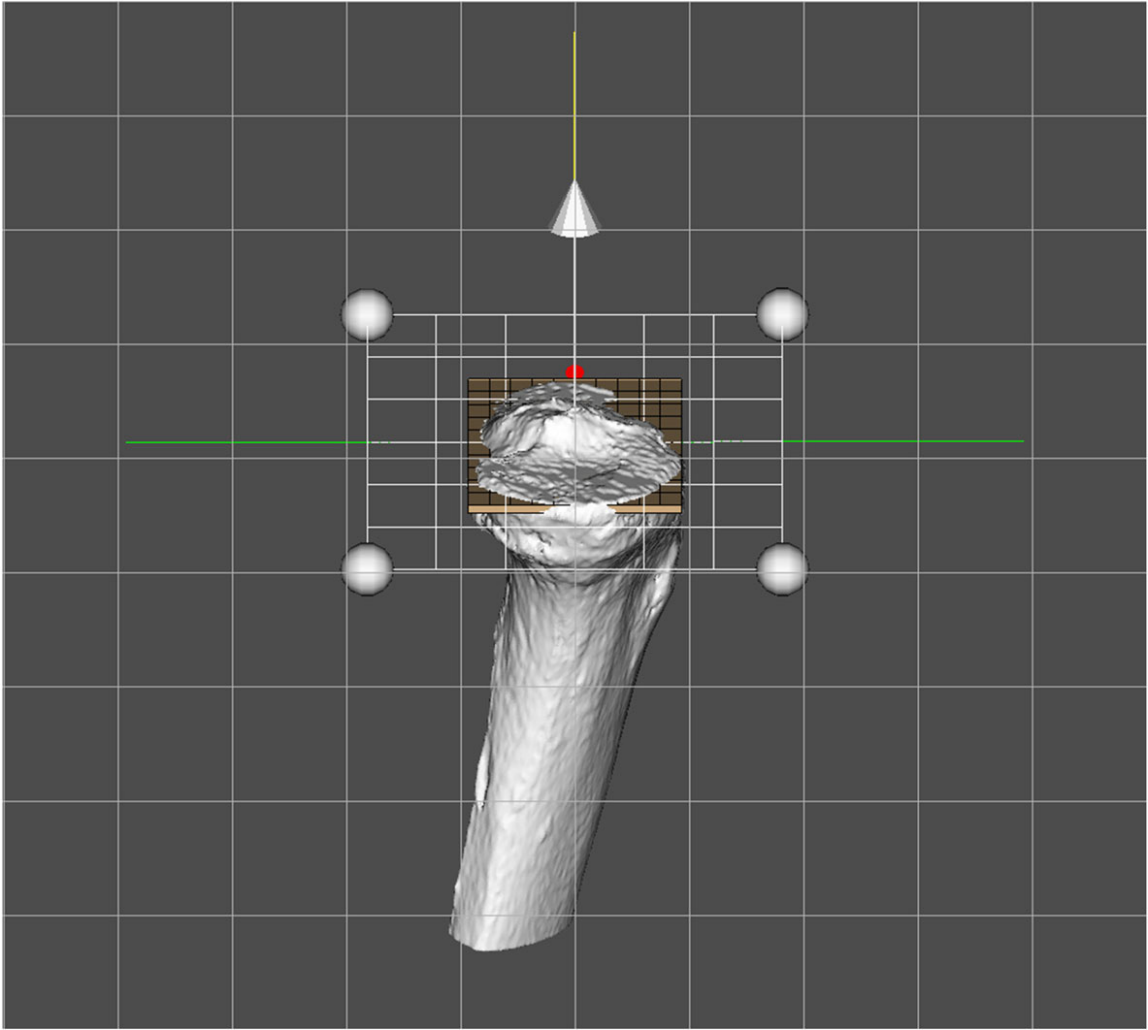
A local coordinate system of the tibia using the CT-derived tibial model. Tibial coordinate system is embedded using a virtual rectangle fitted onto the tibial cross section at the top of the fibular notch level. Then, the fitted rectangle is translated superiorly to the bottom of the medial and lateral tibial plateaus
